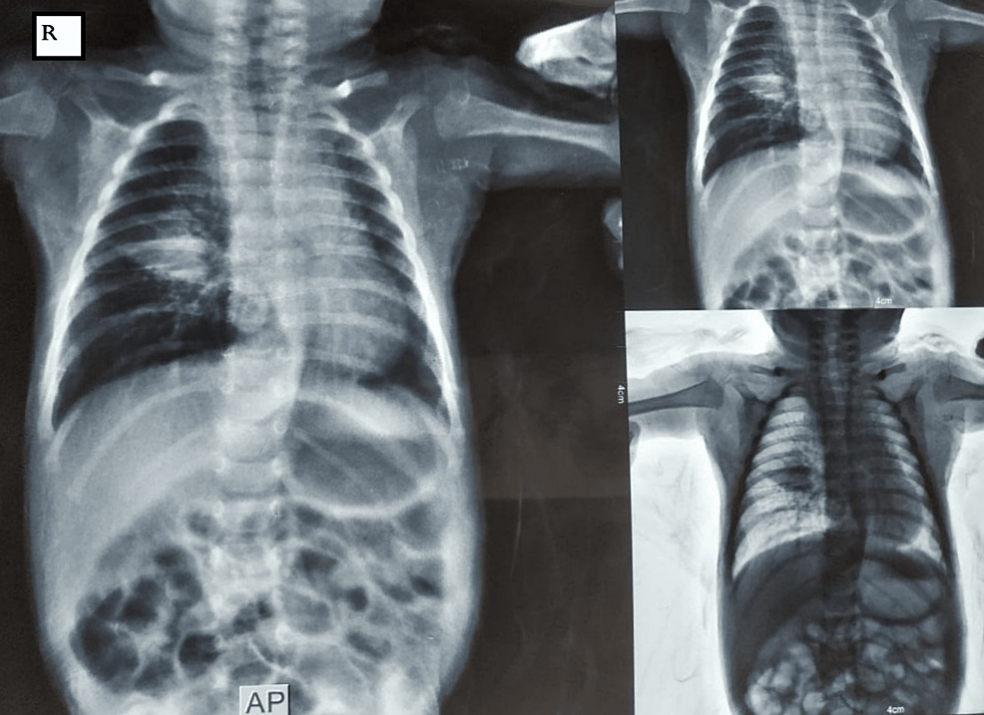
Chest x-ray showing infiltrates on the right upper and lower lobes
